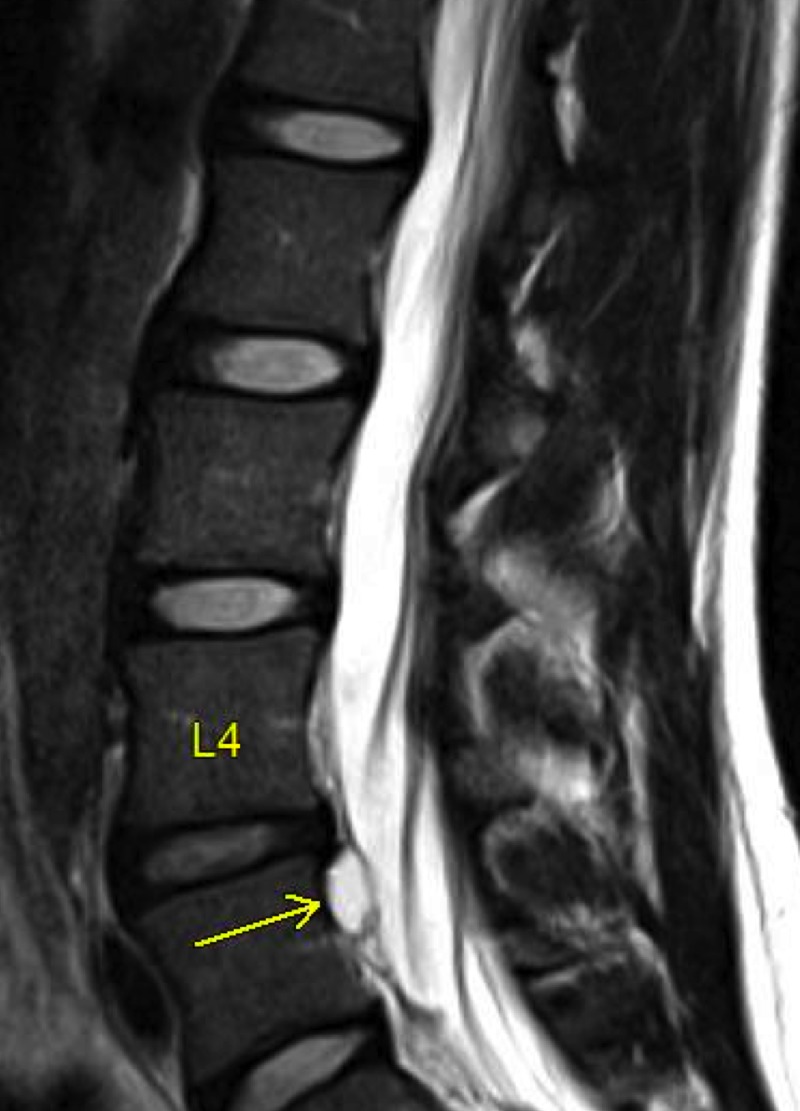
MRI T2 sequence of lumbar spine (sagittal view). Shows a hyperintense cystic lesion behind the L4 vertebral body (arrow).
